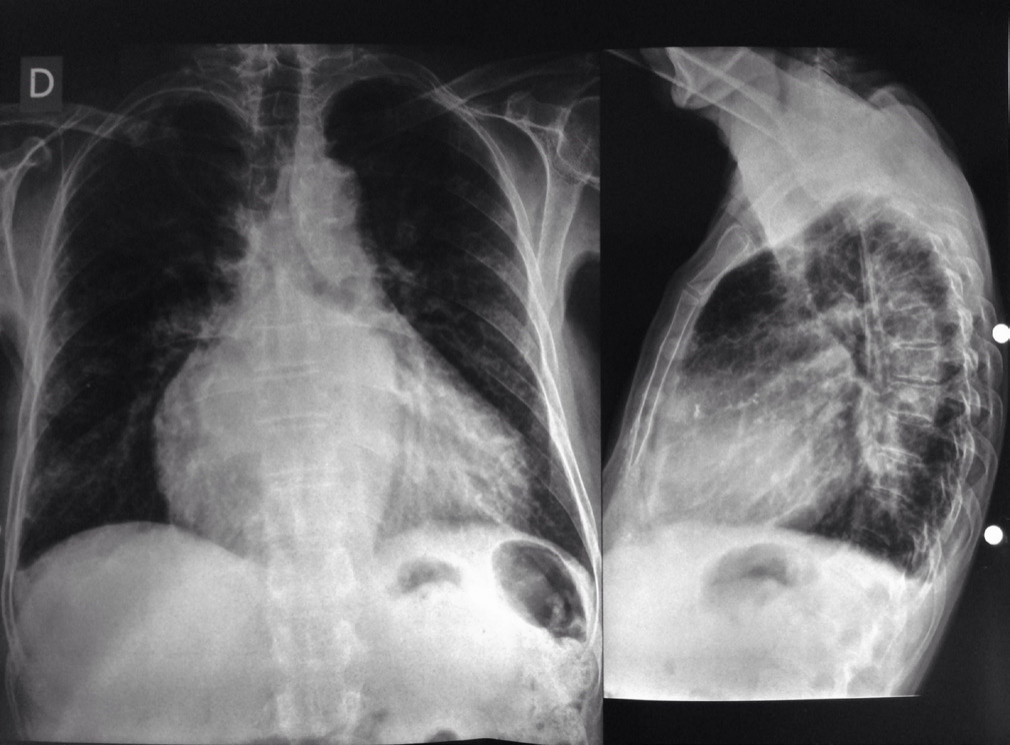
Posterior-anterior (left) and profile (right) chest radiography.
